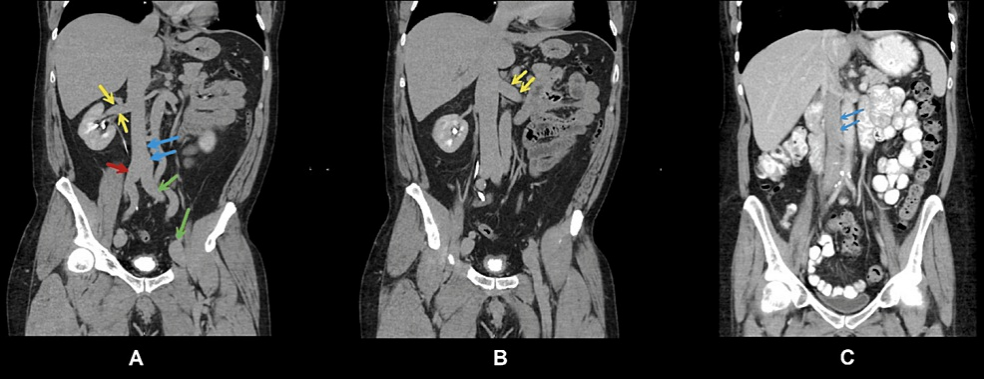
(A) and (B) panels are from our patients: (A) Dilated inferior vena cava (blue arrows), right renal vein (yellow arrows), right common iliac vein (red arrow), left common iliac vein (green arrows); (B) Dilated left renal vein (yellow arrows); (C) Computed tomography of a normal inferior vena cava in a healthy person for comparison. (Panel C) Case courtesy of Andrew Dixon [5]. Permission was obtained from Radiopaedia.org.
